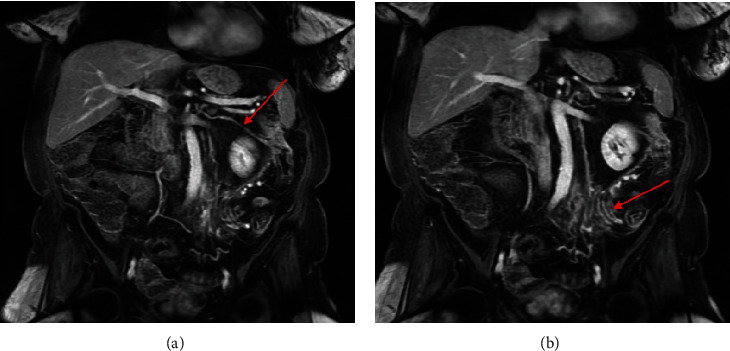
MRA of the abdomen with and without gadolinium 7 weeks after initial presentation. Coronal T1-weighted fat-suppressed VIBE late arterial phase postcontrast images display (a) a prominent marginal artery connecting the middle colic artery to the left colic artery (arrow) and also reveal (b) a cluster of early draining veins in the region of the sigmoid colon mesentery (arrow).
